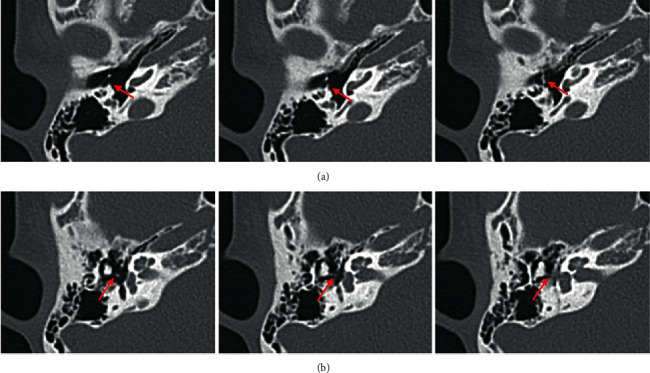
Axial view of preoperative temporal bone CT: (a) a bony bar from the posterior wall of the tympanic cavity connects to the malleus neck (arrow); (b) the long process of the incus is poorly visualized, suggesting incudostapedial disconnection (arrow).
